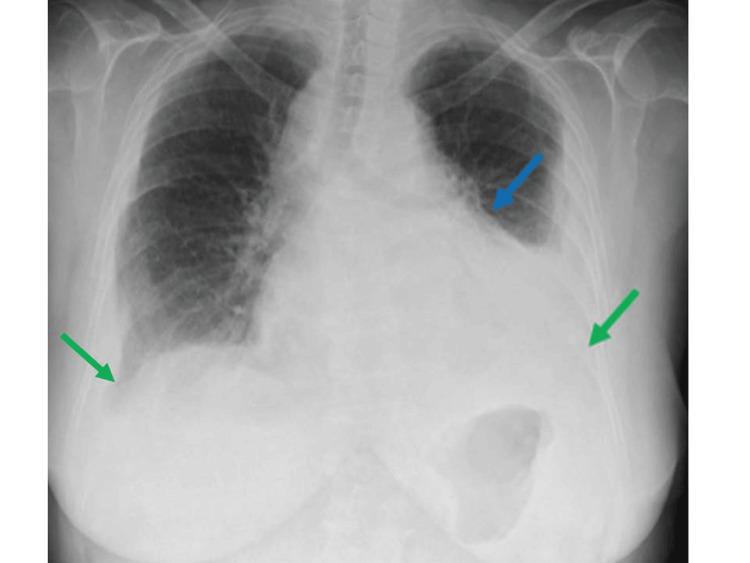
Chest radiograph showing an enlarged cardiac silhouette (blue arrow) and bilateral pleural effusion (green arrows)
